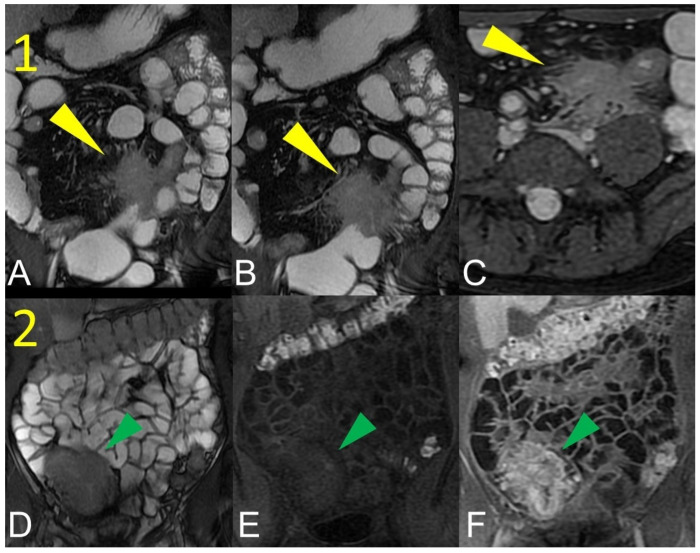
Two different patients with, respectively, an inflammatory conglomerate (Patient 1) of medium size (yellow arrowheads in (A–C)) and of large size (green arrowheads in (D–F)) (Patient 2). Coronal (A,B) and axial (C) fast imaging employing steady-state acquisition (FIESTA) image. Coronal FIESTA image (D) and coronal without (E) and with (F) contrast-enhanced fat-suppressed T1-weighted image. Notably intense contrast enhancement in (F) image underlines the significant state of inflammation of the disease in the active and complicated phase.
